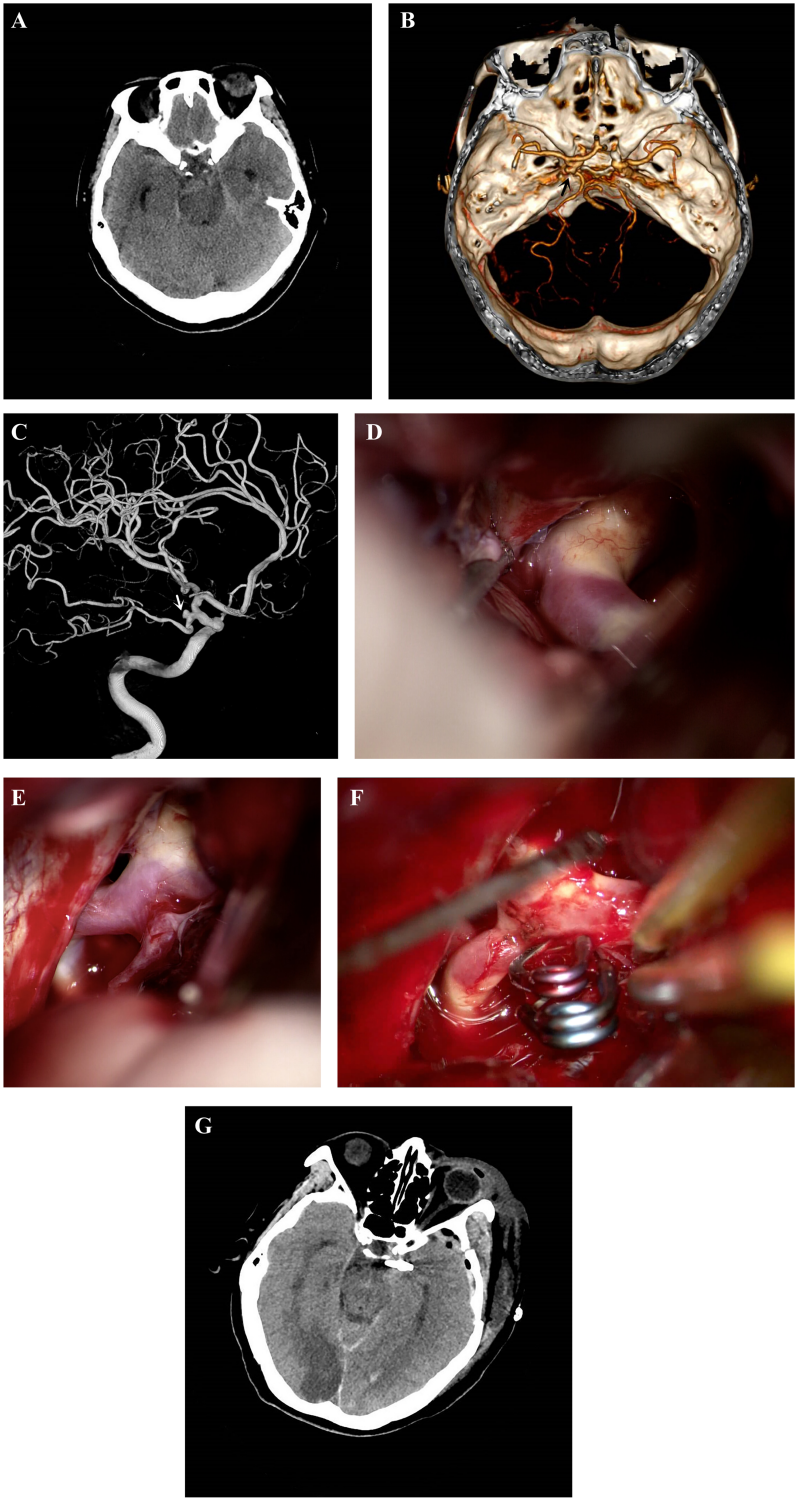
Patient 1. Head CT (A) showed a diffuse SAH in the basilar cistern. CTA (B) revealed the laterally projected PCoA aneurysm (black arrow) on the left side. Preoperative three-dimensional DSA (C) of the left ICA manifested that the aneurysm (white arrow) was about 2.37 × 1.68 mm, arising from the PCoA itself, a few millimeters away from the junction with ICA. Intraoperative observation (D) from the standard pterional approach showed that the greater part of the aneurysm was hidden behind the ICA and the left temporal lobe. The subtemporal approach (E) offers a more lateral line of vision to well visualize the aneurysmal neck and the PCoA. The aneurysm was successfully and safely obliterated with two straight titanium clips (Yasargil titanium clip; No. FT710T and No. FT740T; Aesculap AG, Tuttlingen, Germany) (F). The postoperative head CT (G) disclosed right occipital lobe infarction and no secondary hemorrhage. CT, computed tomography; CTA, computed tomography angiography; DSA, digital subtraction angiography; ICA, internal carotid artery; PCoA, posterior communicating artery; PCA, posterior cerebral artery; SAH, subarachnoid hemorrhage.
